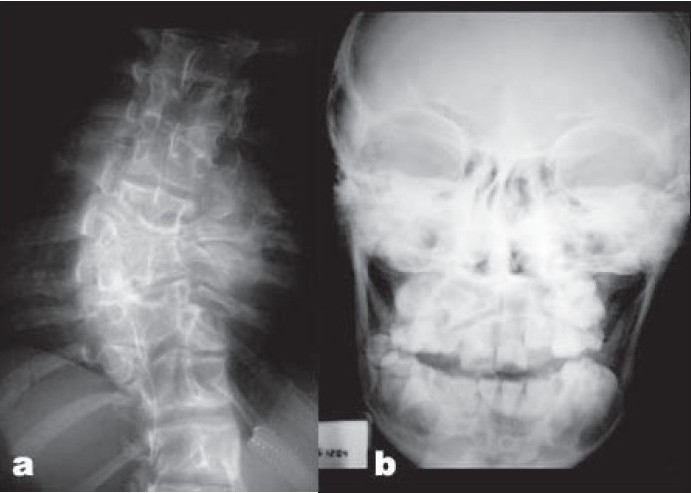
Case 2 (a) Dorsal spine X-ray film showing scoliosis, block vertebrae and hemivertebrae. (b) Skull X-ray film showing crowded teeth
